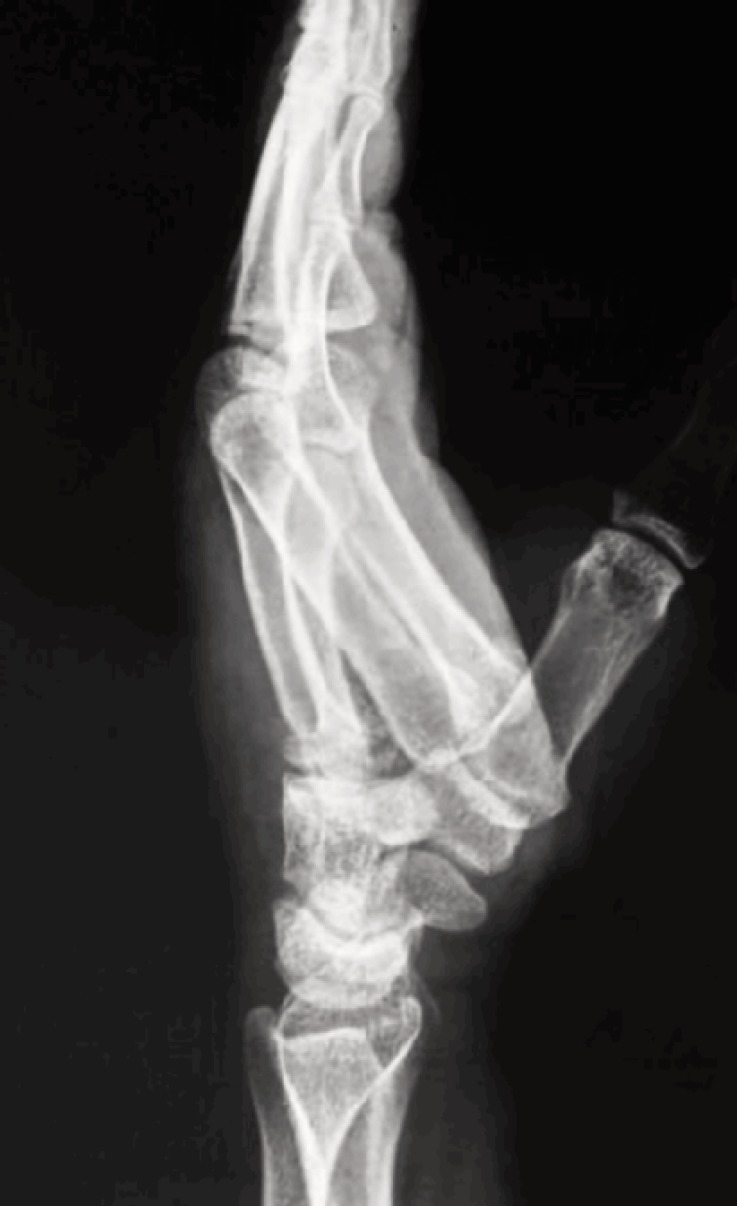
Lateral radiograph showing Classification Type B. Volar dislocation of index and middle finger metacarpals without any associated fractures.
